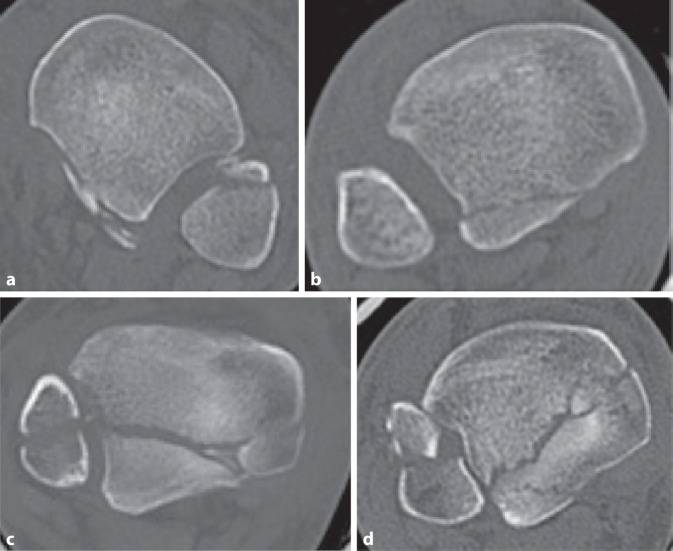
Die Auswertung der CT erfolgte in der axialen Ebene 5 mm proximal der tibialen Gelenkfläche sowie in der sagittalen Ebene 1 cm medial der Inzisur. Die Definition der verschiedenen Frakturtypen basierte auf den hypothetischen Unfallmechanismen. Als Ursache für Mason-Typ-1-Frakturen definierten sie eine Avulsion des posterioren inferioren tibiofibularen Ligaments, bedingt durch auf den plantar-flektierten, entlasteten Fuß wirkende Rotationskräfte. Als Unfallmechanismus der Mason-Typ-2A-Frakturen identifizierten sie den Anprall des rotierenden Talus auf die tibiale Gelenkfläche, bedingt durch die auf den belasteten Fuß (Neutralstellung oder Plantarflexion) wirkenden Rotationskräfte. Bei weiterer Rotation des Talus kam es nach ihrer Definition zu einem zweiten posteromedialen Fragment, in der Regel in einem 45°-Winkel, welches sie als Mason Typ 2B klassifizierten. Mason-Typ-3-Frakturen sahen sie verursacht durch eine rein axiale Lastübertragung des Talus bei plantar-flektiertem Fuß. Begleitverletzungen der tibialen Gelenkfläche, des Malleolus medialis (knöchern und ligamentär), der Fibula sowie der Syndesmose wurden ebenfalls erfasst und ausgewertet. Die Interobserver-Reliabilität (2 Observer) zeigte mit einem Cohens-κ-Koeffizienten von 0,919 eine hohe Übereinstimmung [27].
